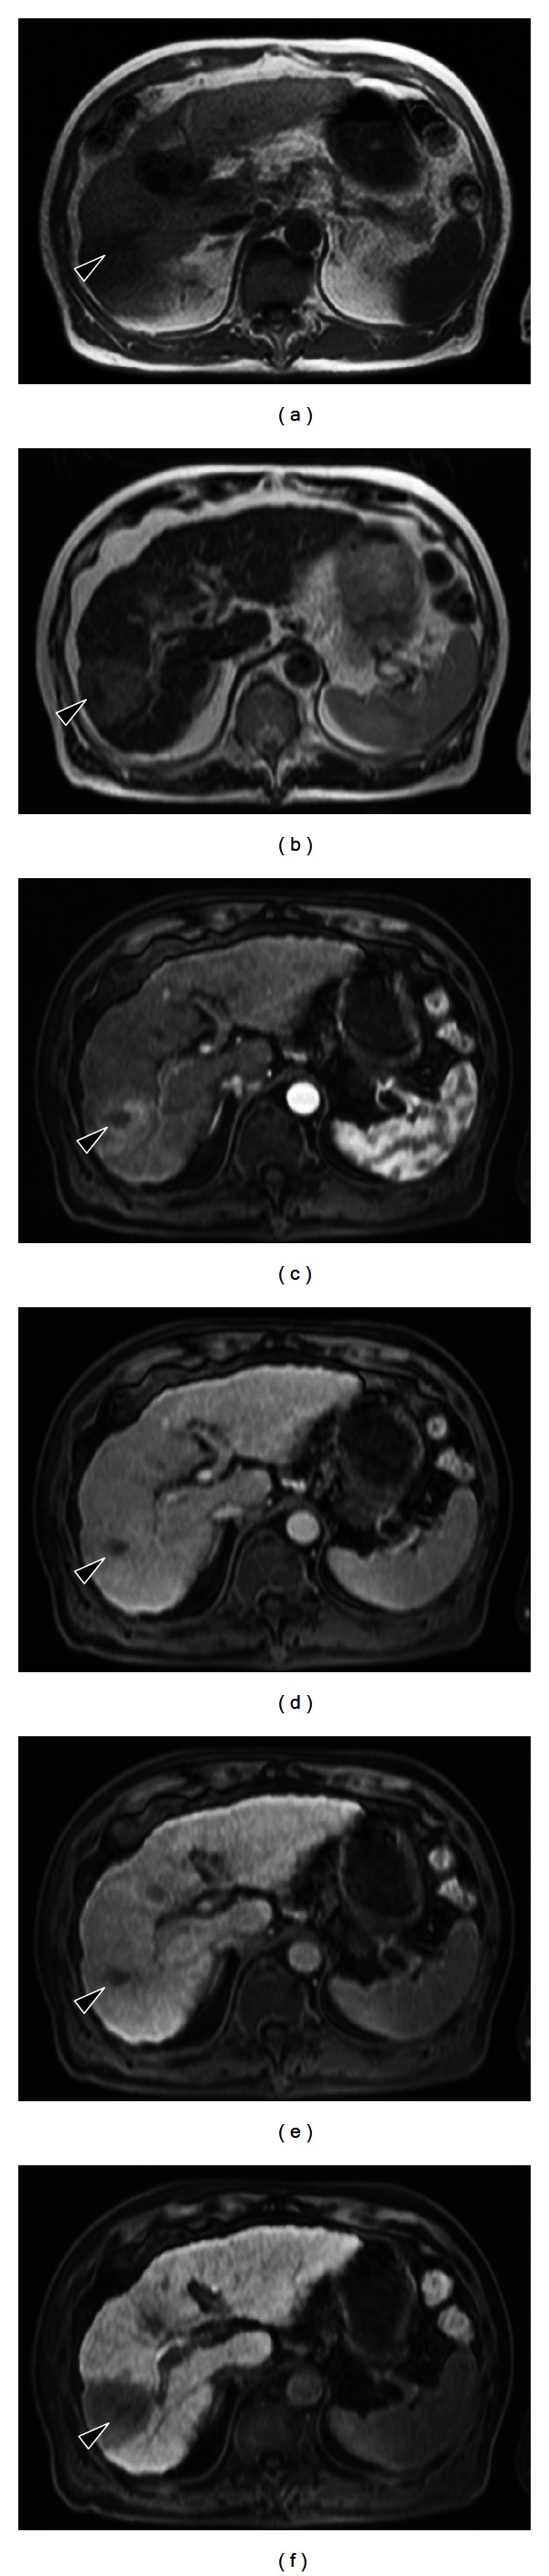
MR images obtained approximately two months after transcatheter arterial chemoembolization and radiofrequency ablation. (a) A hypodense area measuring approximately 3 cm in diameter is observed on T1-weighted image. (b) A hyperintense area is found on T2-weighted image. ((c)–(f)) Gadolinium ethoxybenzyl diethylenetriamine pentaacetic acid-enhanced images. In the arterial phase following contrast injection (c), the center of the tumor is enhanced intensely and homogeneously. In the delayed phase (d) and hepatobiliary phase (f), the tumor is completely washed out. e: portal phase.
